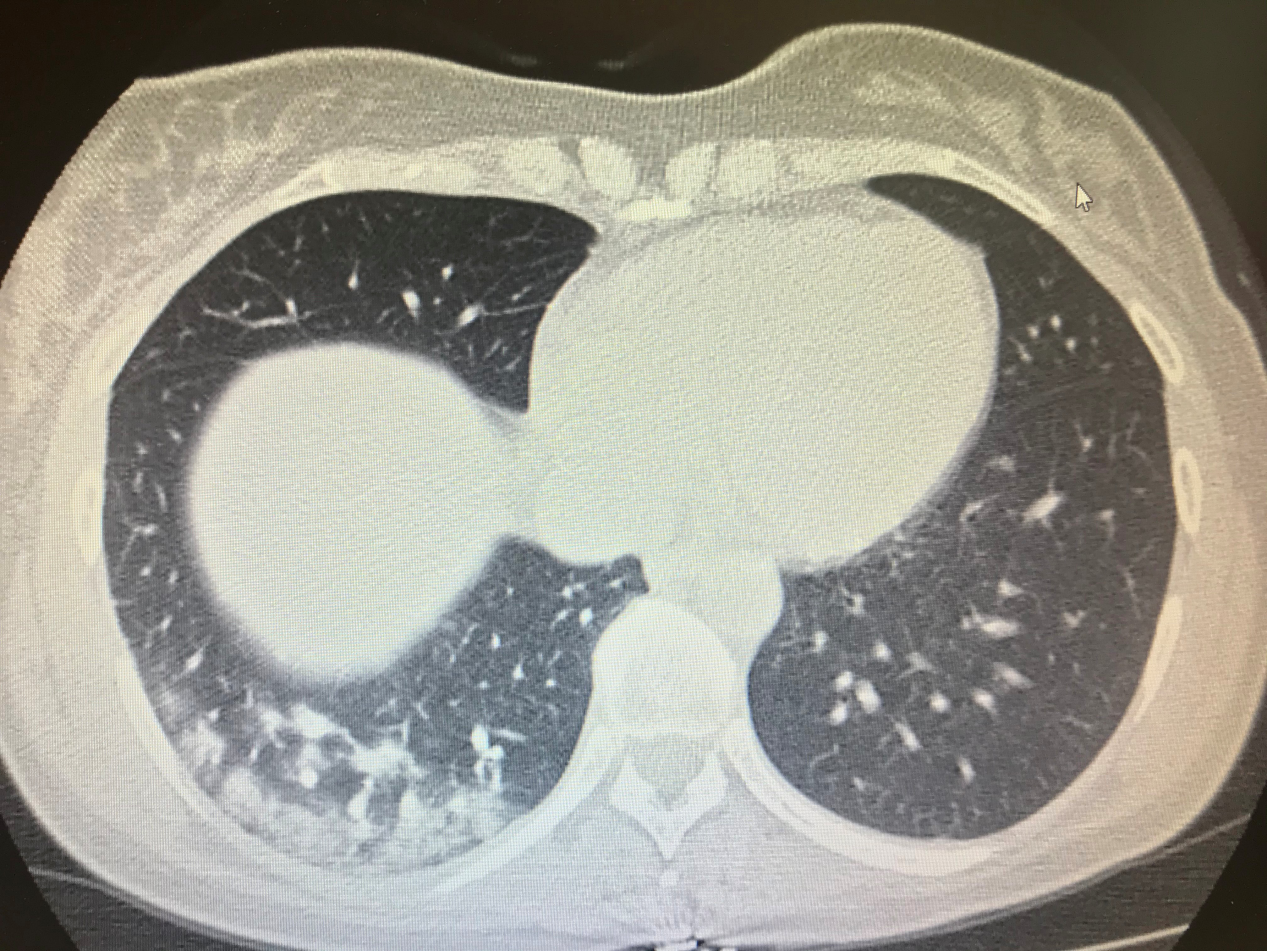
Lung CT scan with evidence of multifocal pneaumonia.
